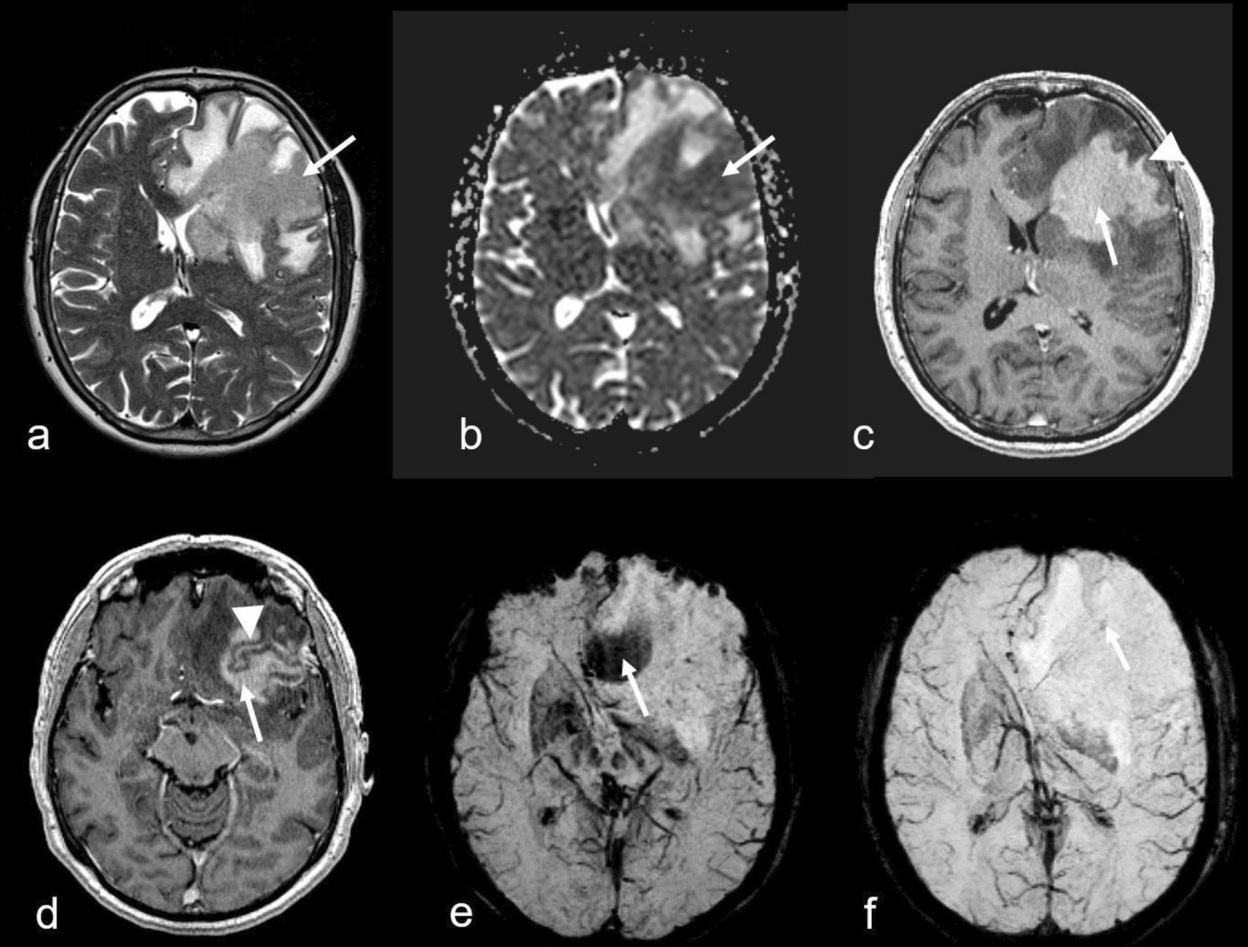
69-year-old woman presenting with progressive non-fluent aphasia caused by primary large B-cell lymphoma of immune-privileged sites (IP-LBCL), negative for Epstein–Barr virus. MRI reveals a large left-sided frontal lesion with associated midline shift (a: T2-weighted imaging (WI); arrow), inhomogeneous restricted diffusion (b: apparent diffusion coefficient map; mean ± SD: 0.75 ± 0.12 × 10⁻^3^ mm^2^/s; arrow), homogeneous contrast enhancement (c, d: T1WI; arrows) sparing the cortex (c, d; arrowheads), and focal areas of different signal loss patterns on susceptibility-WI (e, f; arrow)
